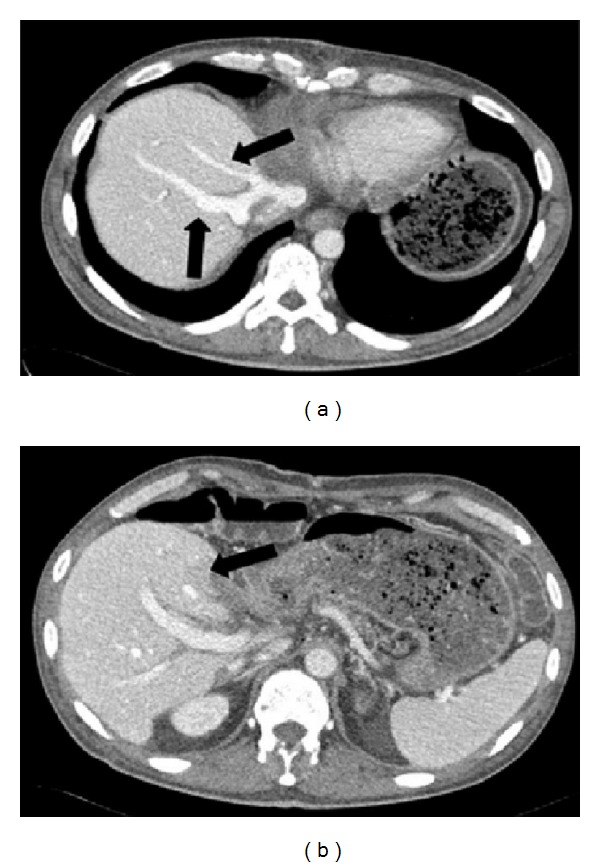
CT scan of combined heart-right liver transplantation: arrows of Figure 2(a) show middle and right hepatic veins; arrow of Figure 2(b) shows the segment IV of the liver with normal perfusion.
